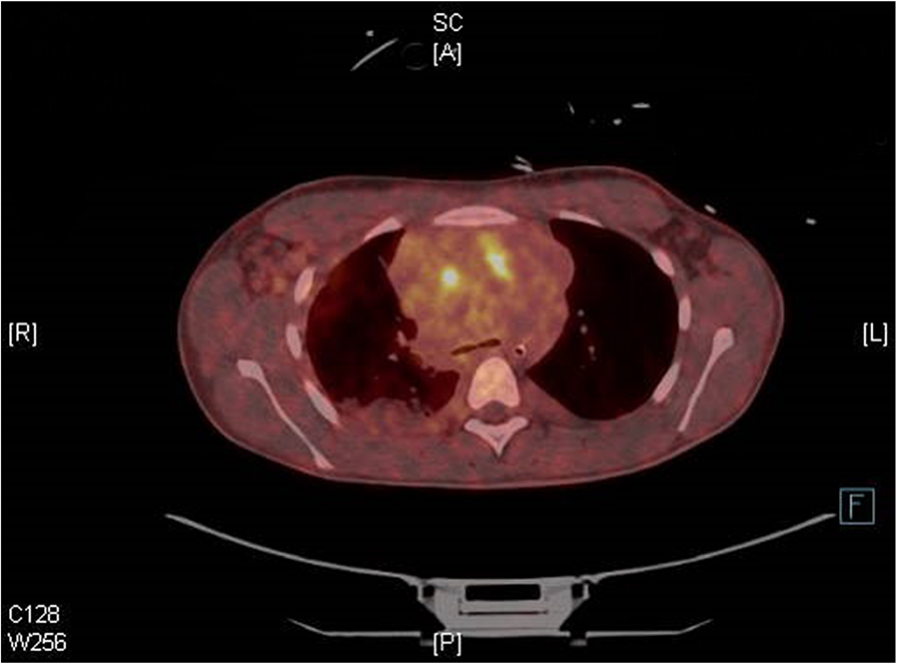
Positron emission tomography computed tomography scan of mediastinum. Increased activity equaling the mediastinal tumor mass.
